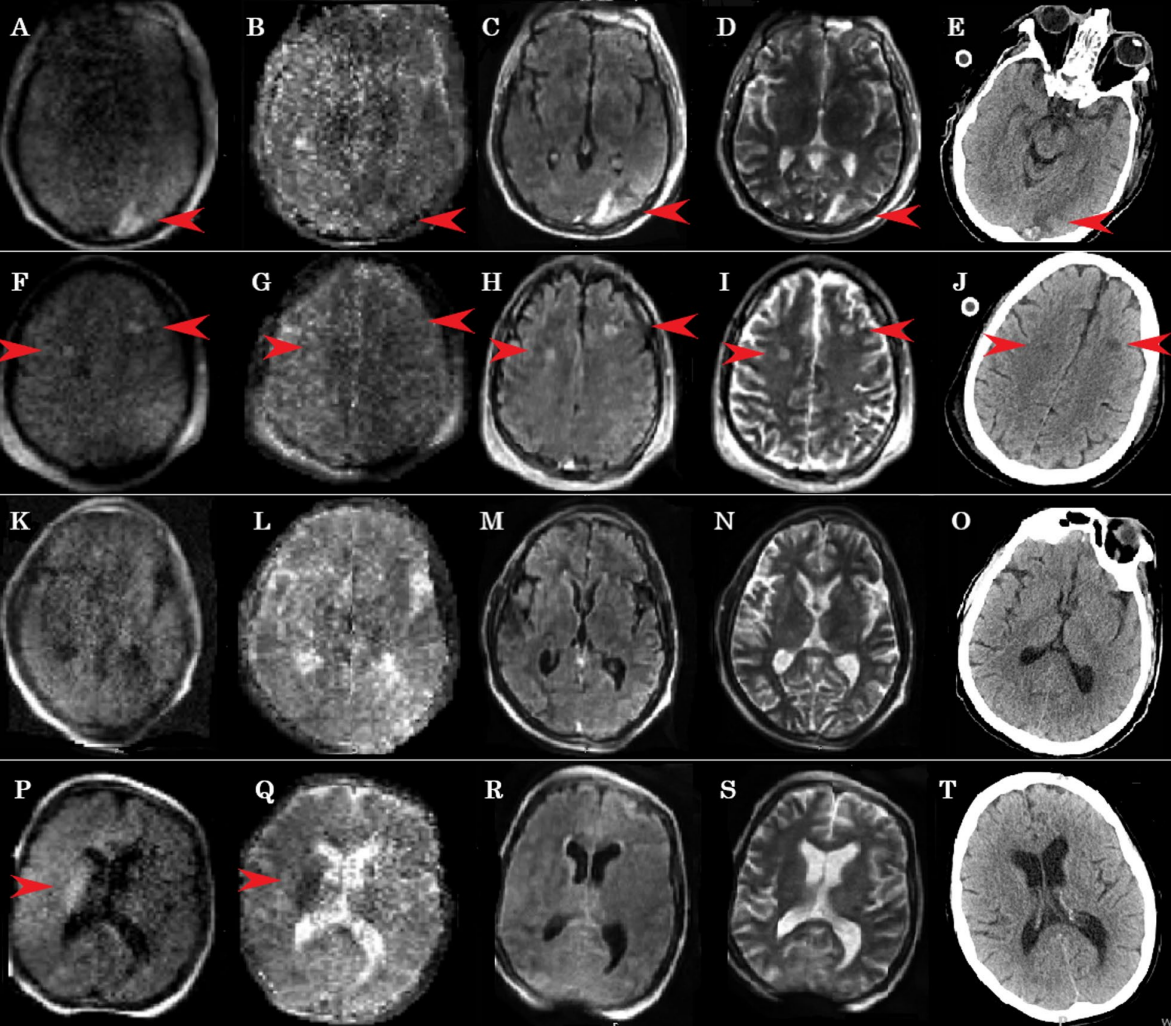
Point-of-care brain MRI images and corresponding CT images. Images A, F, K, P are diffusion-weighted imaging MRI, images B, G, L, Q are apparent diffusion coefficient MRI, images C, H, M, R are fluid-attenuated inversion recovery MRI, and images E, J, O, T are CT scans. Patient 1 (Images A–J) demonstrated acute occipital stroke with hemorrhagic conversion as well as two acute frontal lobe strokes are denoted by red arrows. Patient 2 (K–O) had normal MRI and CT scan. Patient 3 (P–T) had an acute right basal ganglia stroke (red arrow) confirmed on other MRI sequences and not identified on CT. Entire series of MRI sequences and images for these patients are available as the link to Digital Imaging and Communications in Medicine (DICOM) in the Hyperfine Cloud (Additional file 1: Appendix)
